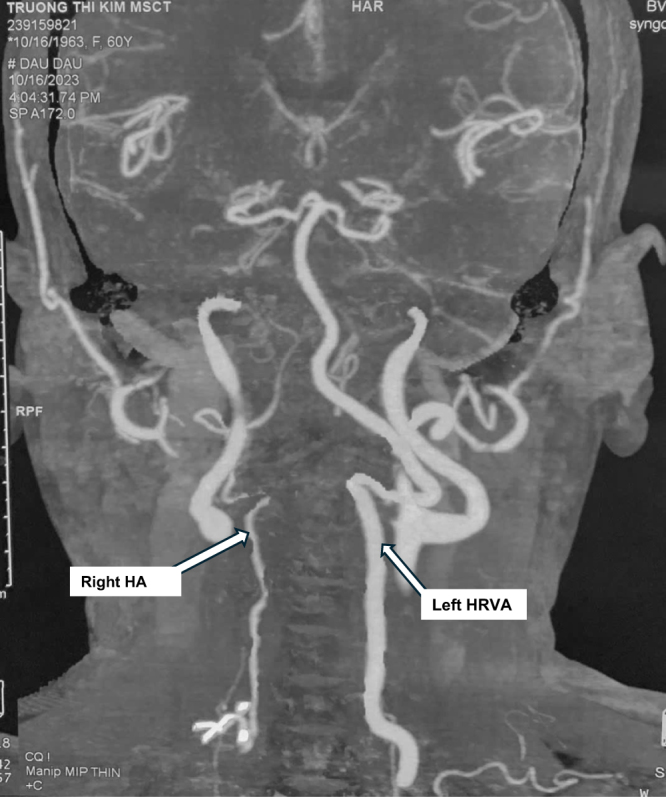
CT angiography demonstrated right hypoplasia of vertebral artery (HVA) with diameter of 1.5 mm and left HRVA.
